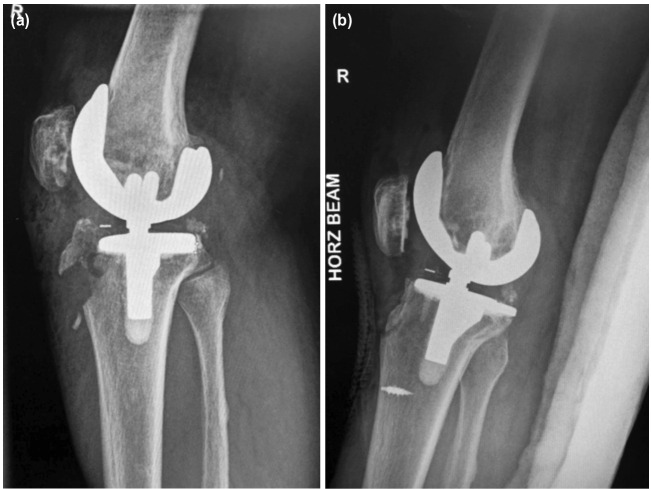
(a) Pre-operative, (b) post-operative radiograph lateral view of right knee (metallic anchor suture used here).
